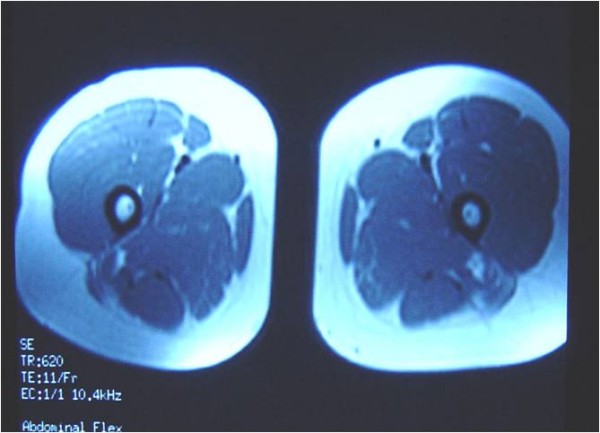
Horizontal MRI section six weeks later.
